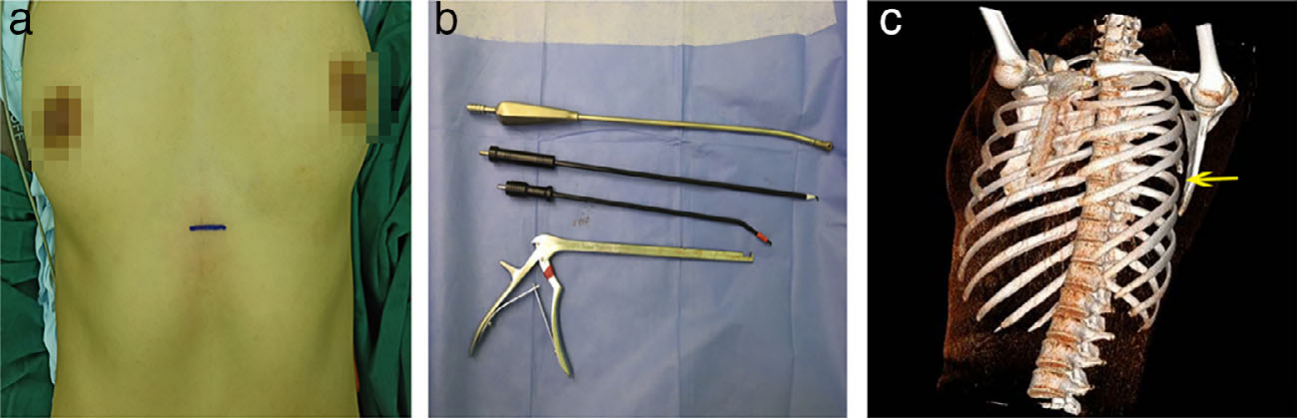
Intraoperative photographs. (a) Subxiphoid incision. (b) Instruments used to perform uniportal subxiphoid video‐assisted thoracic costectomy. (c) Postoperative chest computed tomography image.
